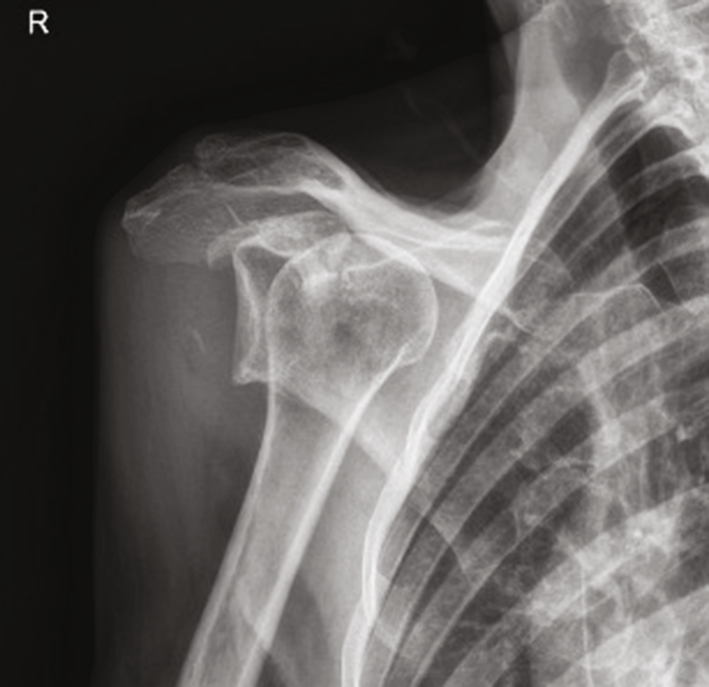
Pre-reduction Grashey radiograph of the right shoulder demonstrating anterior dislocation of the humeral head. No associated fracture is seen.
